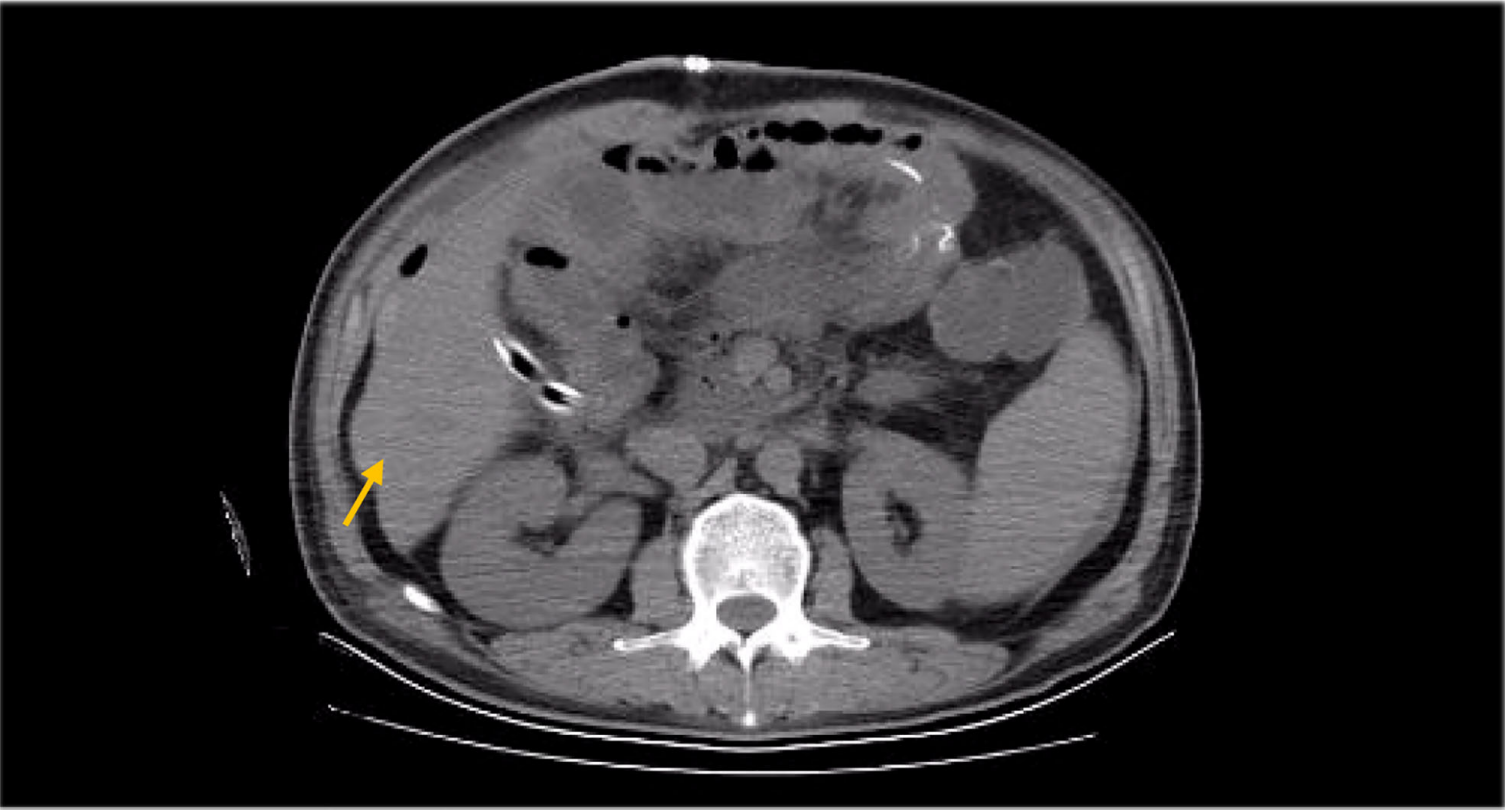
CT findings 1 month after surgery (no liver metastasis).
